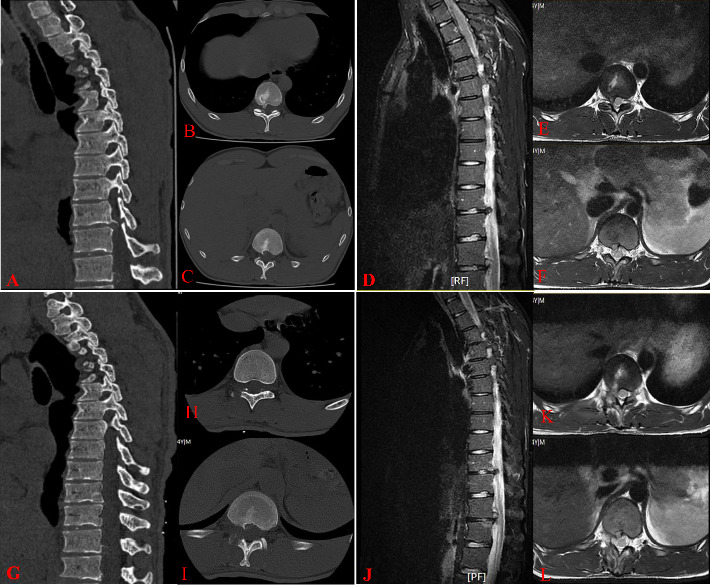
Preoperative CT and MRI scans (A–F) and postoperative follow-up CT and MRI scans (G–L).
